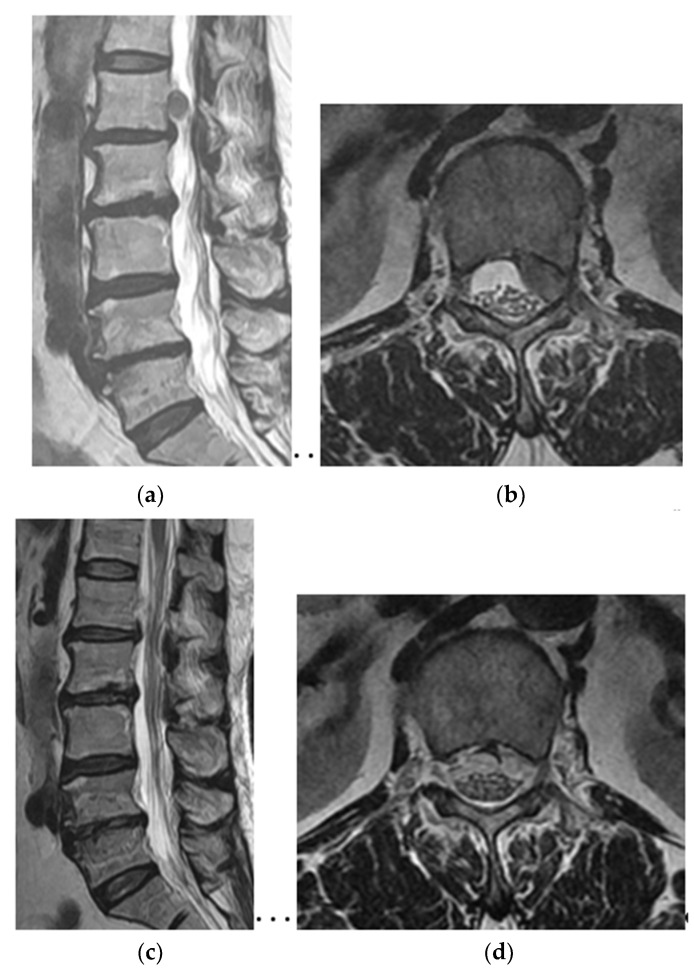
A 47-year-old man complained of radiating pain in the anterior thigh of the left leg. Preoperative MRI showed left-sided upward migrated disc herniation at L1–L2 (a,b). Ruptured disc fragments were removed using the fully endoscopic transforaminal approach (video S1). Although a small remnant disc particle was suspicious on postoperative MRI (c,d), radicular pain of left leg completely disappeared.
